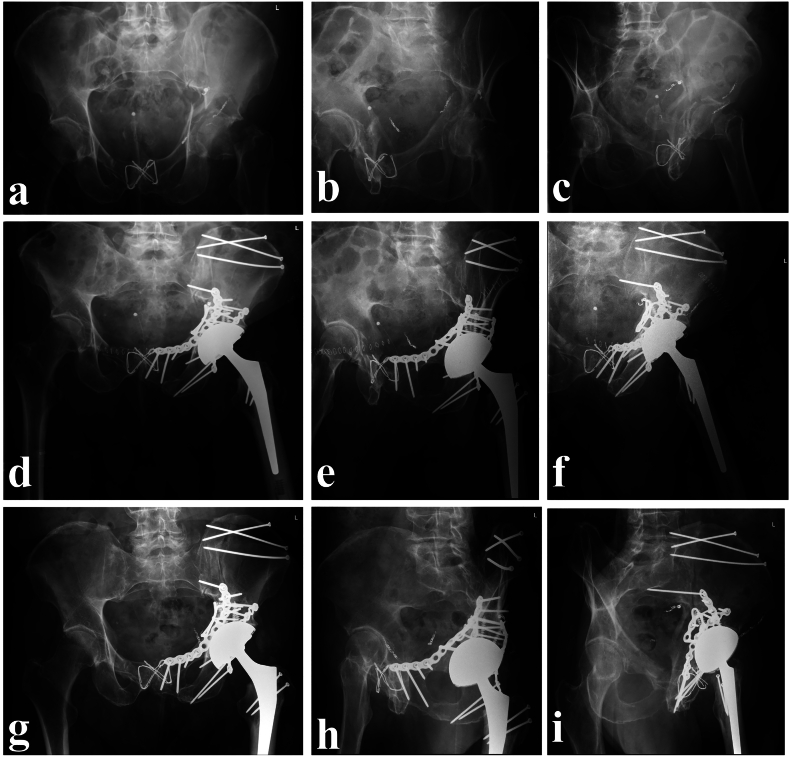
Case 1; (a-c) Pelvis a.-p., obturator, and ala radiographs preoperatively demonstrate the extent of the displacement of the quadrilateral plate and medialization of the femoral head and the femoral neck fracture; (d-f) in a staged procedure, ORIF of the acetabulum and hip arthroplasty were performed at two and nine days post trauma; (g-i) at the six month follow-up, the patient was ambulating without crutches, living independently at home and without the need for analgesics.
